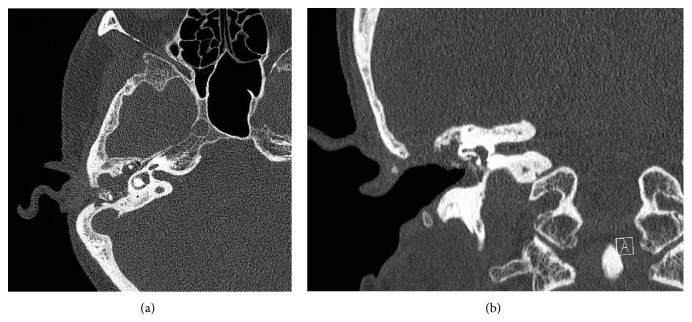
(a) High resolution CT axial image at the level of the lateral semicircular canal demonstrates a fluid filled mastoid cavity, partial erosion of incus, and an intact anterior epitympanic air cell partitioned by the cog. (b) High resolution coronal CT of right temporal bone demonstrating an approximate 9 mm defect involving the superiomedial external ear canal and tegmen mastoideum.
